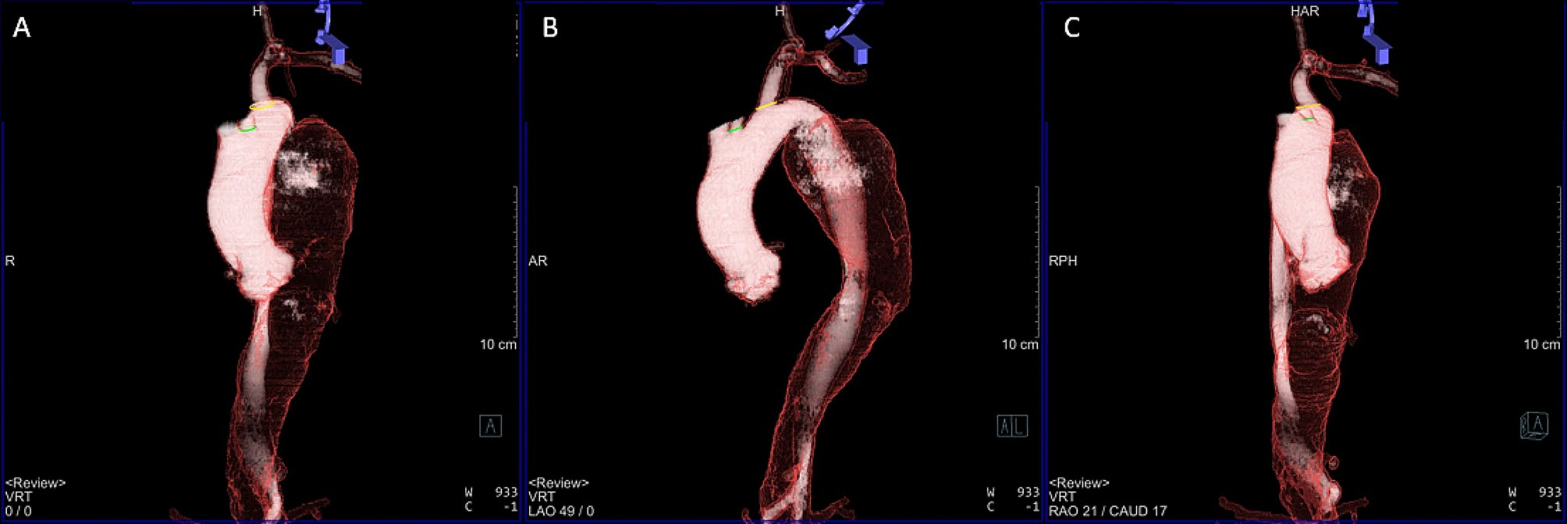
3D reconstruction of CTA with landmarks of ostia in the (A) anteroposterior view and (B) arch view, showing the optimum working angle with full visualization of LSA and LCCA, and in the (C) en face view to visualize the proximal shape of LSA. The white solid area indicates the true lumen, and the transparent area indicates the false lumen
